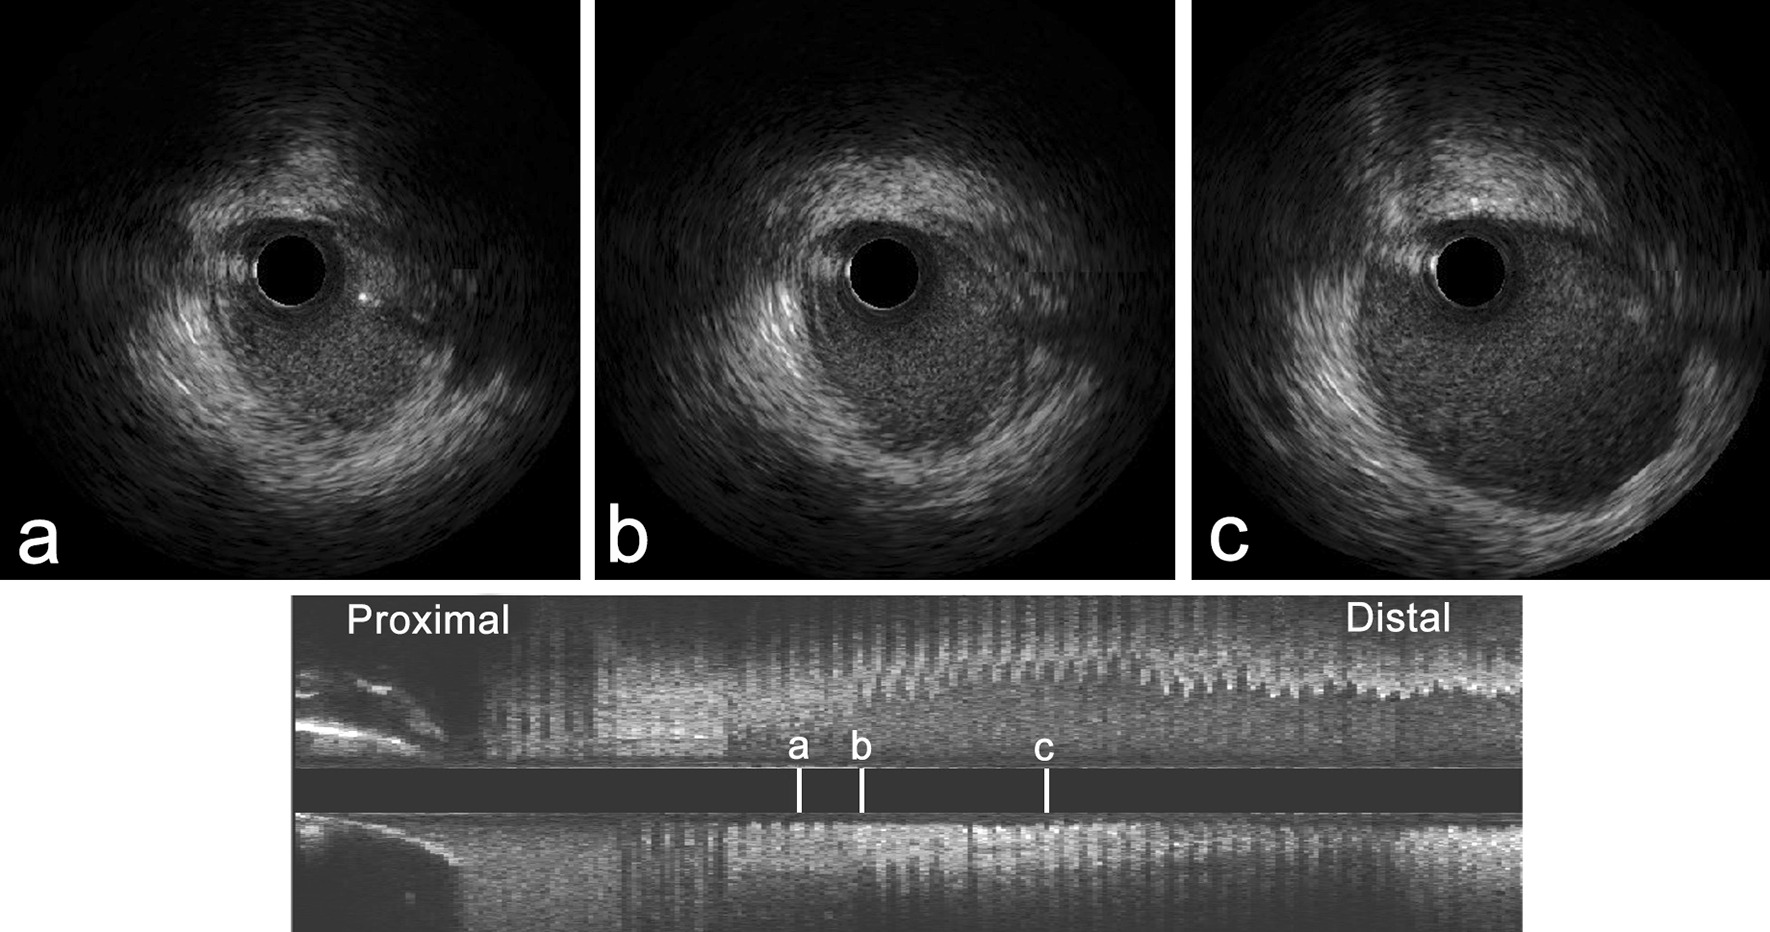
Intravenous ultrasound (IVUS) images. After the first percutaneous transluminal coronary angioplasty (PTCA), IVUS demonstrated that the external elastic membrane (EEM) areas of the ostium, proximal segment and middle segment of the LMCA were 7.11 (a), 8.34 (b) and 15.68 (c), respectively (in mm^2^). It also showed negative remodelling of the ostium of the LMCA (remodelling index = 0.45). The remodelling index was calculated by the ratio of the EEM areas of the middle LMCA segment to the LMCA ostium (7.11 mm^2^/15.68 mm^2^)
